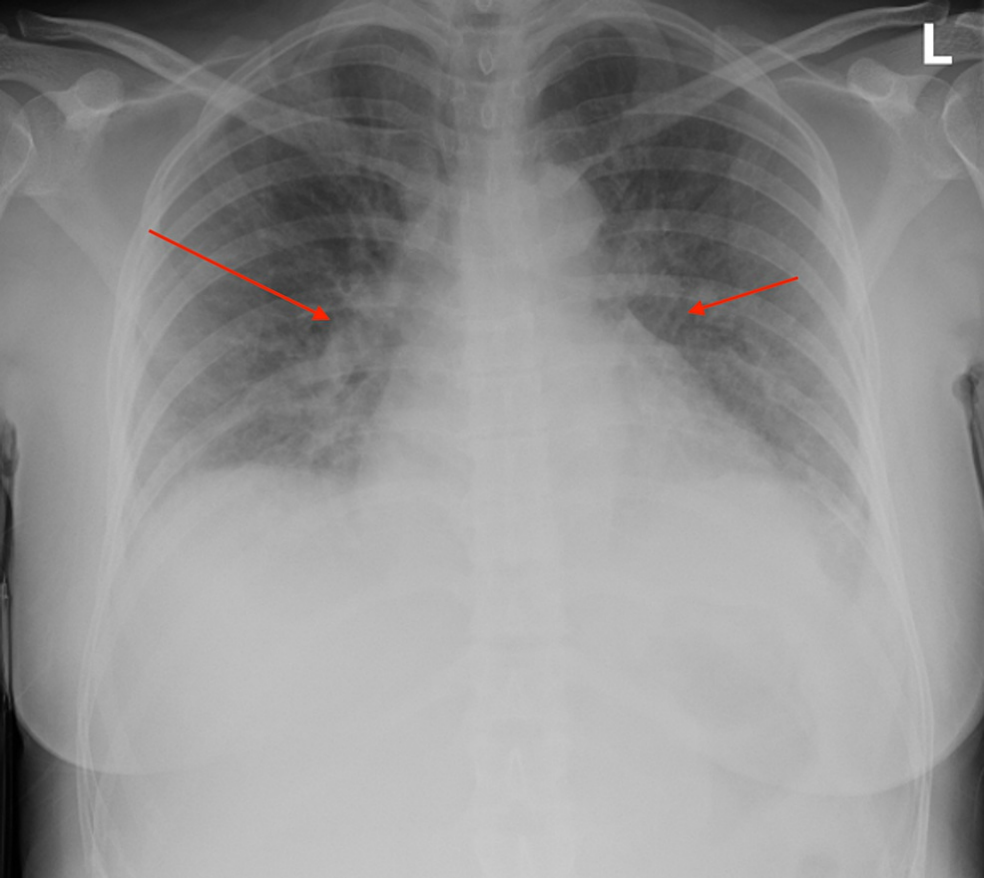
Chest X-ray showing bilateral interstitial edema.
